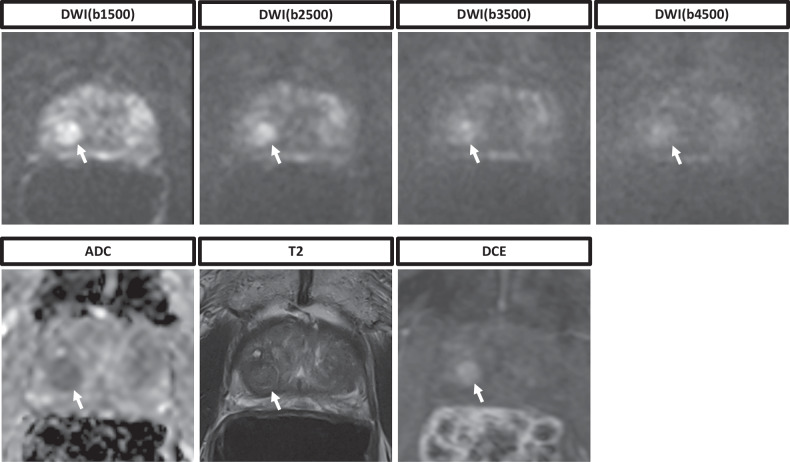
Representative images of a 79-year-old patient. The patient presented with a suspicious digital rectal exam while having a normal prostate-specific antigen of 2.5 ng/mL. The whole multiparametric MRI protocol is shown, consisting of T2-weighted sequences, DWI sequences with calculation of the ADC map, and DCE sequences. The heterogeneous lesion in the posterior transition zone shows a mostly encapsulated nodule that initially was rated by all readers with a PI-RADS score of 3 due to the additional diffusion restriction in both reading protocols that included either the DWI(b1500) or DWI(b2500). However, the diagnostic confidence declined with higher *b-*values. ADC, Apparent diffusion coefficient; DCE, Dynamic contrast enhanced; DWI, Diffusion-weighted imaging; MRI, Magnetic resonance imaging; PI-RADS, Prostate Imaging Reporting and Data System
